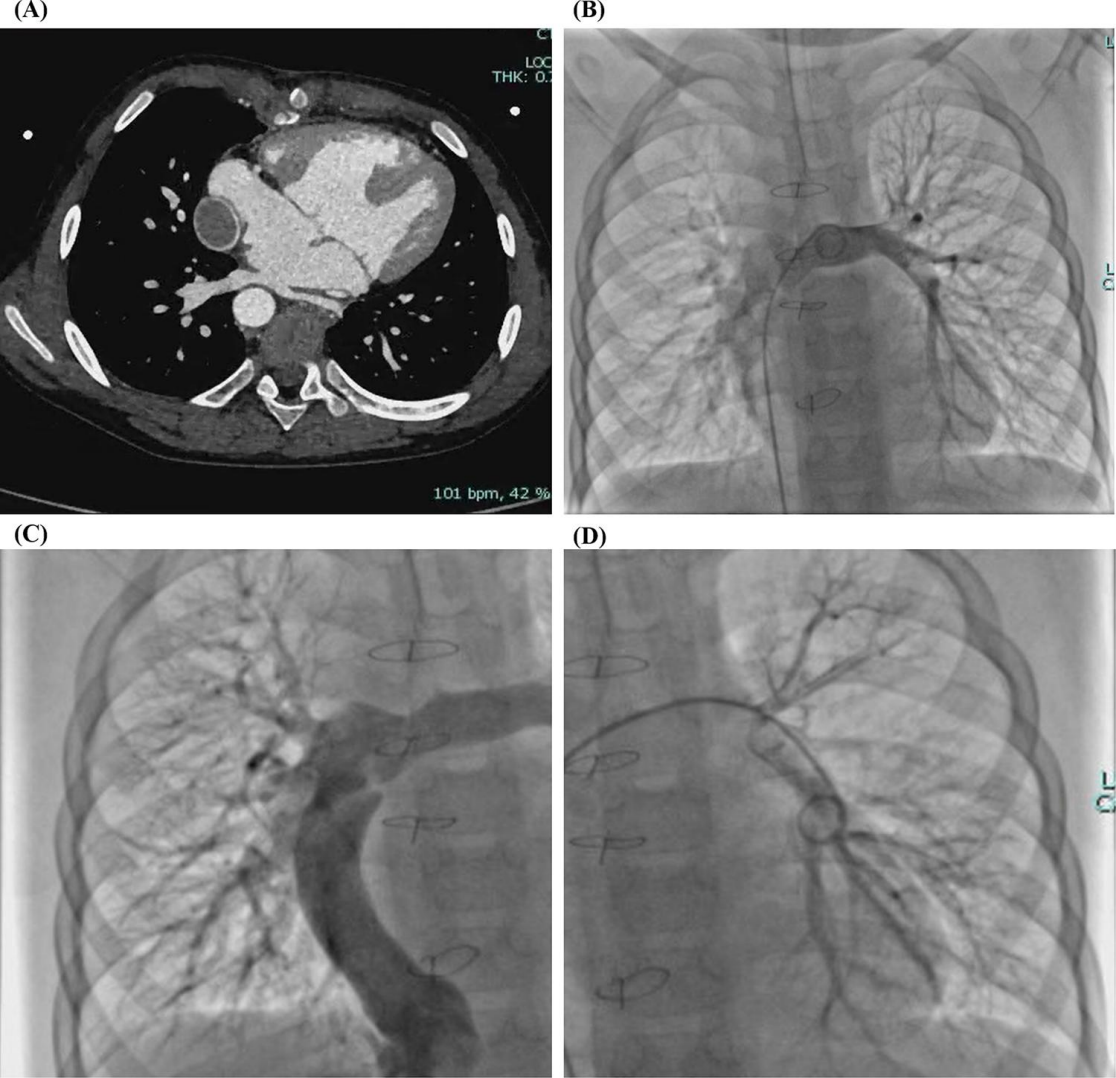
(A) Contrast-enhanced cardiac CT after total cavopulmonary connection (TCPC) confirming complete disappearance of the bilateral diffuse pulmonary arteriovenous malformations (PAVMs). (B–D) Cardiac catheterization after TCPC demonstrating complete resolution of bilateral diffuse PAVMs.
